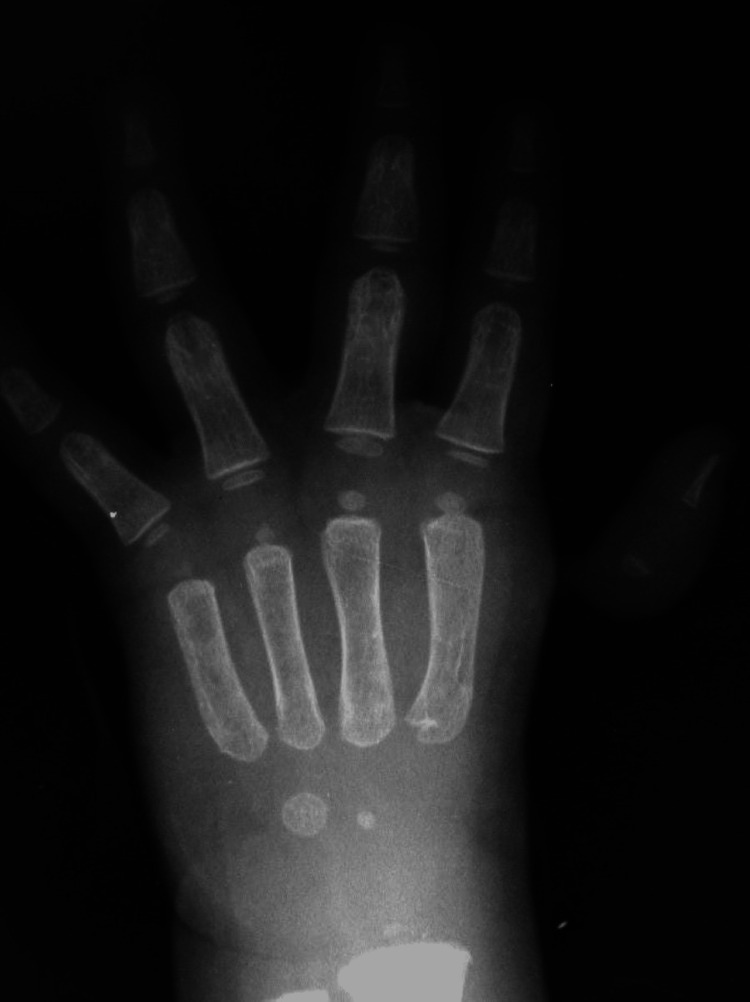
X-ray anterior posterior (AP) view of right hand showing right thumb hypoplasia.
